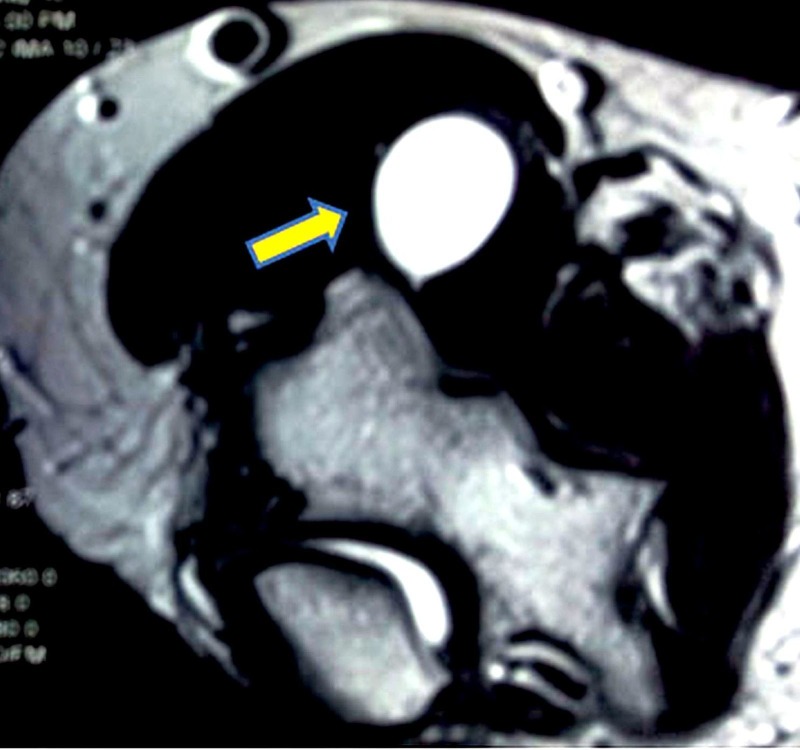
MRI (T2 weighted) cross section view showing the cyst in the anterolateral aspect of the elbow
